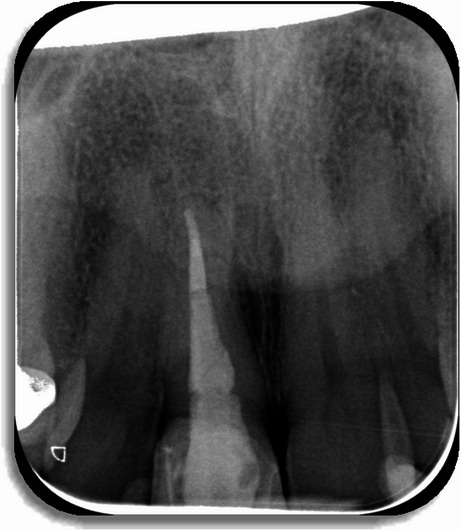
Six-month follow-up PA radiograph indicating apical area healing
